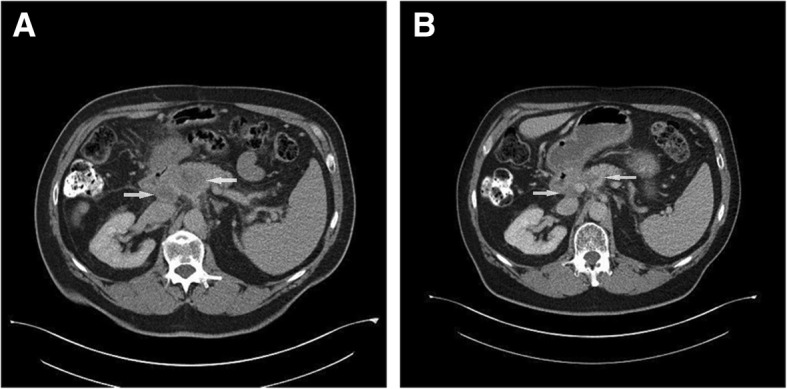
Evolution of periampullary adenopathies on reintroduction of regorafenib. A In january 2017 periampullary adenopathies were seen on follow-up CT-scan. B CT-scan after 6 months of treatment with regorafenib shows a partial response
